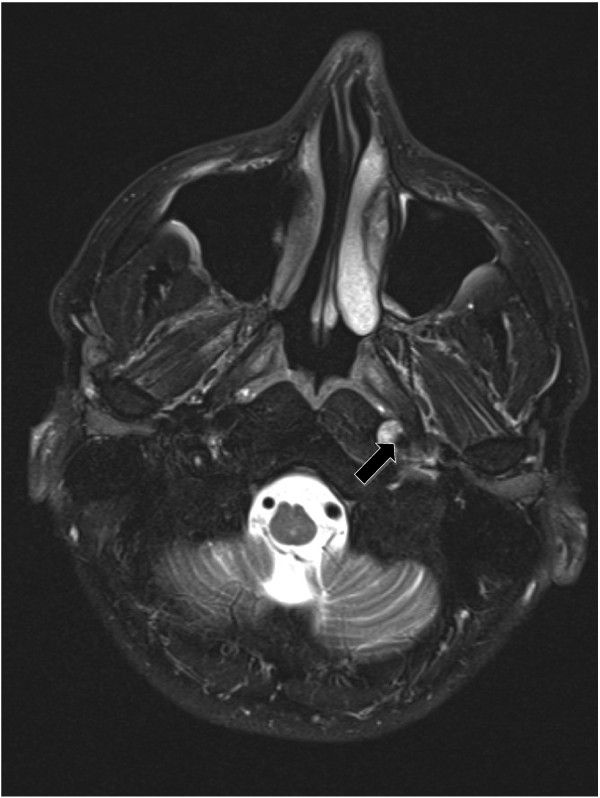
T2-weighted MRI showing a left sided non-occlusive dissection extending to the level of the petrous segment (arrow).
